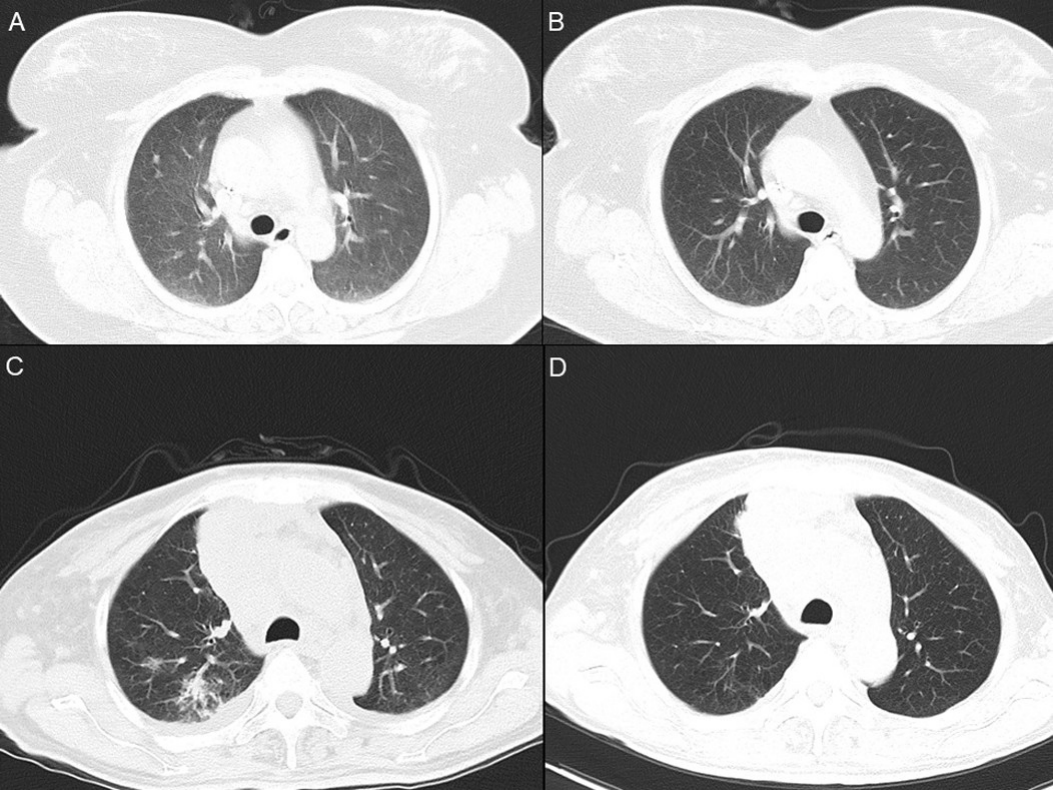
Thoracic computed tomography scan of Case 2 and 3 before and after specific antibiotic treatment. Panel A: Case 2, day 9 after admission, showing diffuse interstitial infiltrates and ground-glass nodules in both lungs; Panel B: Case 2, day 40 after admission, showing clearance of infiltrates and nodules; Panel C: Case 3, day 5 after admission, showing infiltrates in the posterior segment of the upper lobe of the right lung; Panel D: Case 3, day 64 after admission, showing clearance infiltrates.
